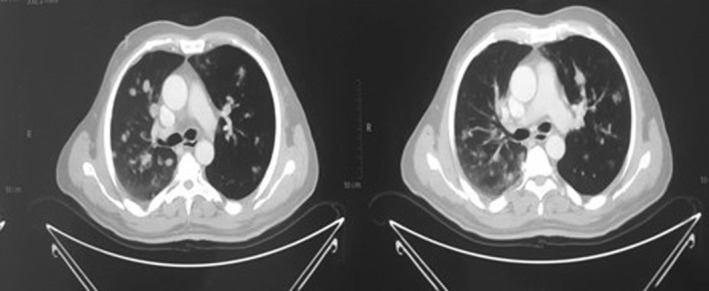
CT chest with metastasis from primary leiomyosarcoma of thyroid. Metastasis mark red.
